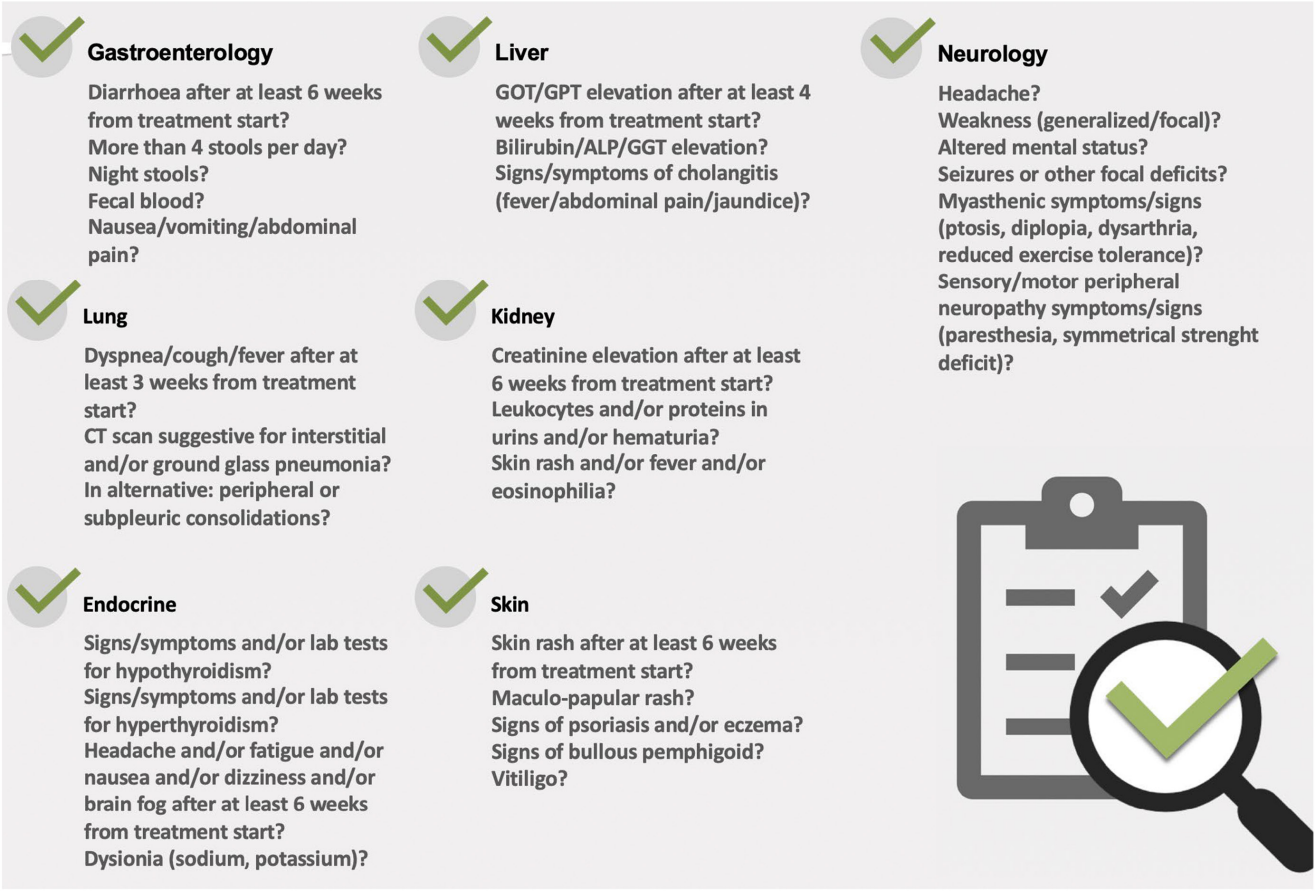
Operative checklist for suspected irAEs. ALP, alkaline phosphatase; CT scan, computed tomography scan; GGT, gamma‐glutamyl transferase; GOT/GPT, liver transaminases.
